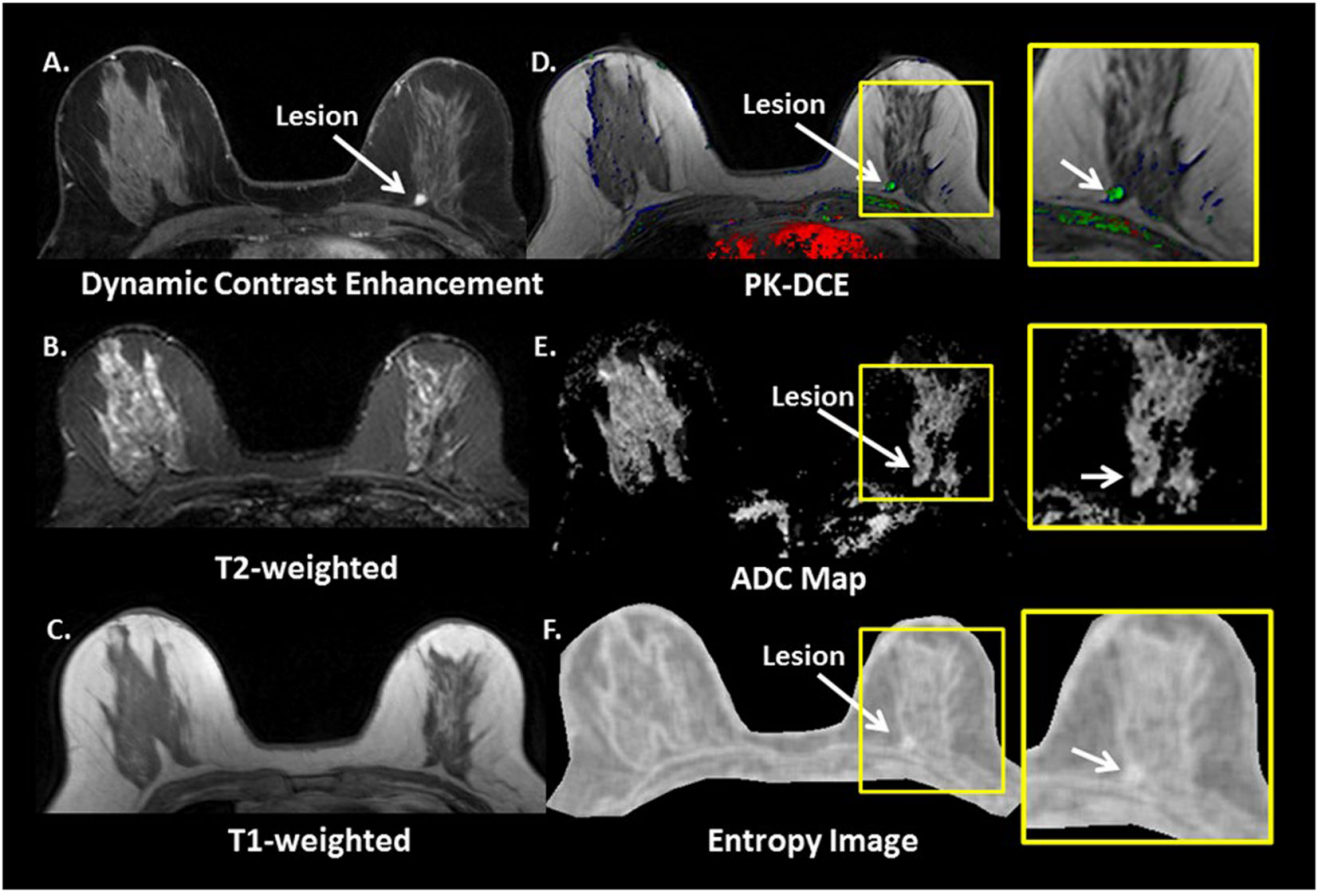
Typical multiparametric breast image of a benign patient. a Dynamic contract enhanced, b T2-weighted, c T1-weighted, d Pharmacokinetic-DCE (PK-DCE) overlay of *K*
